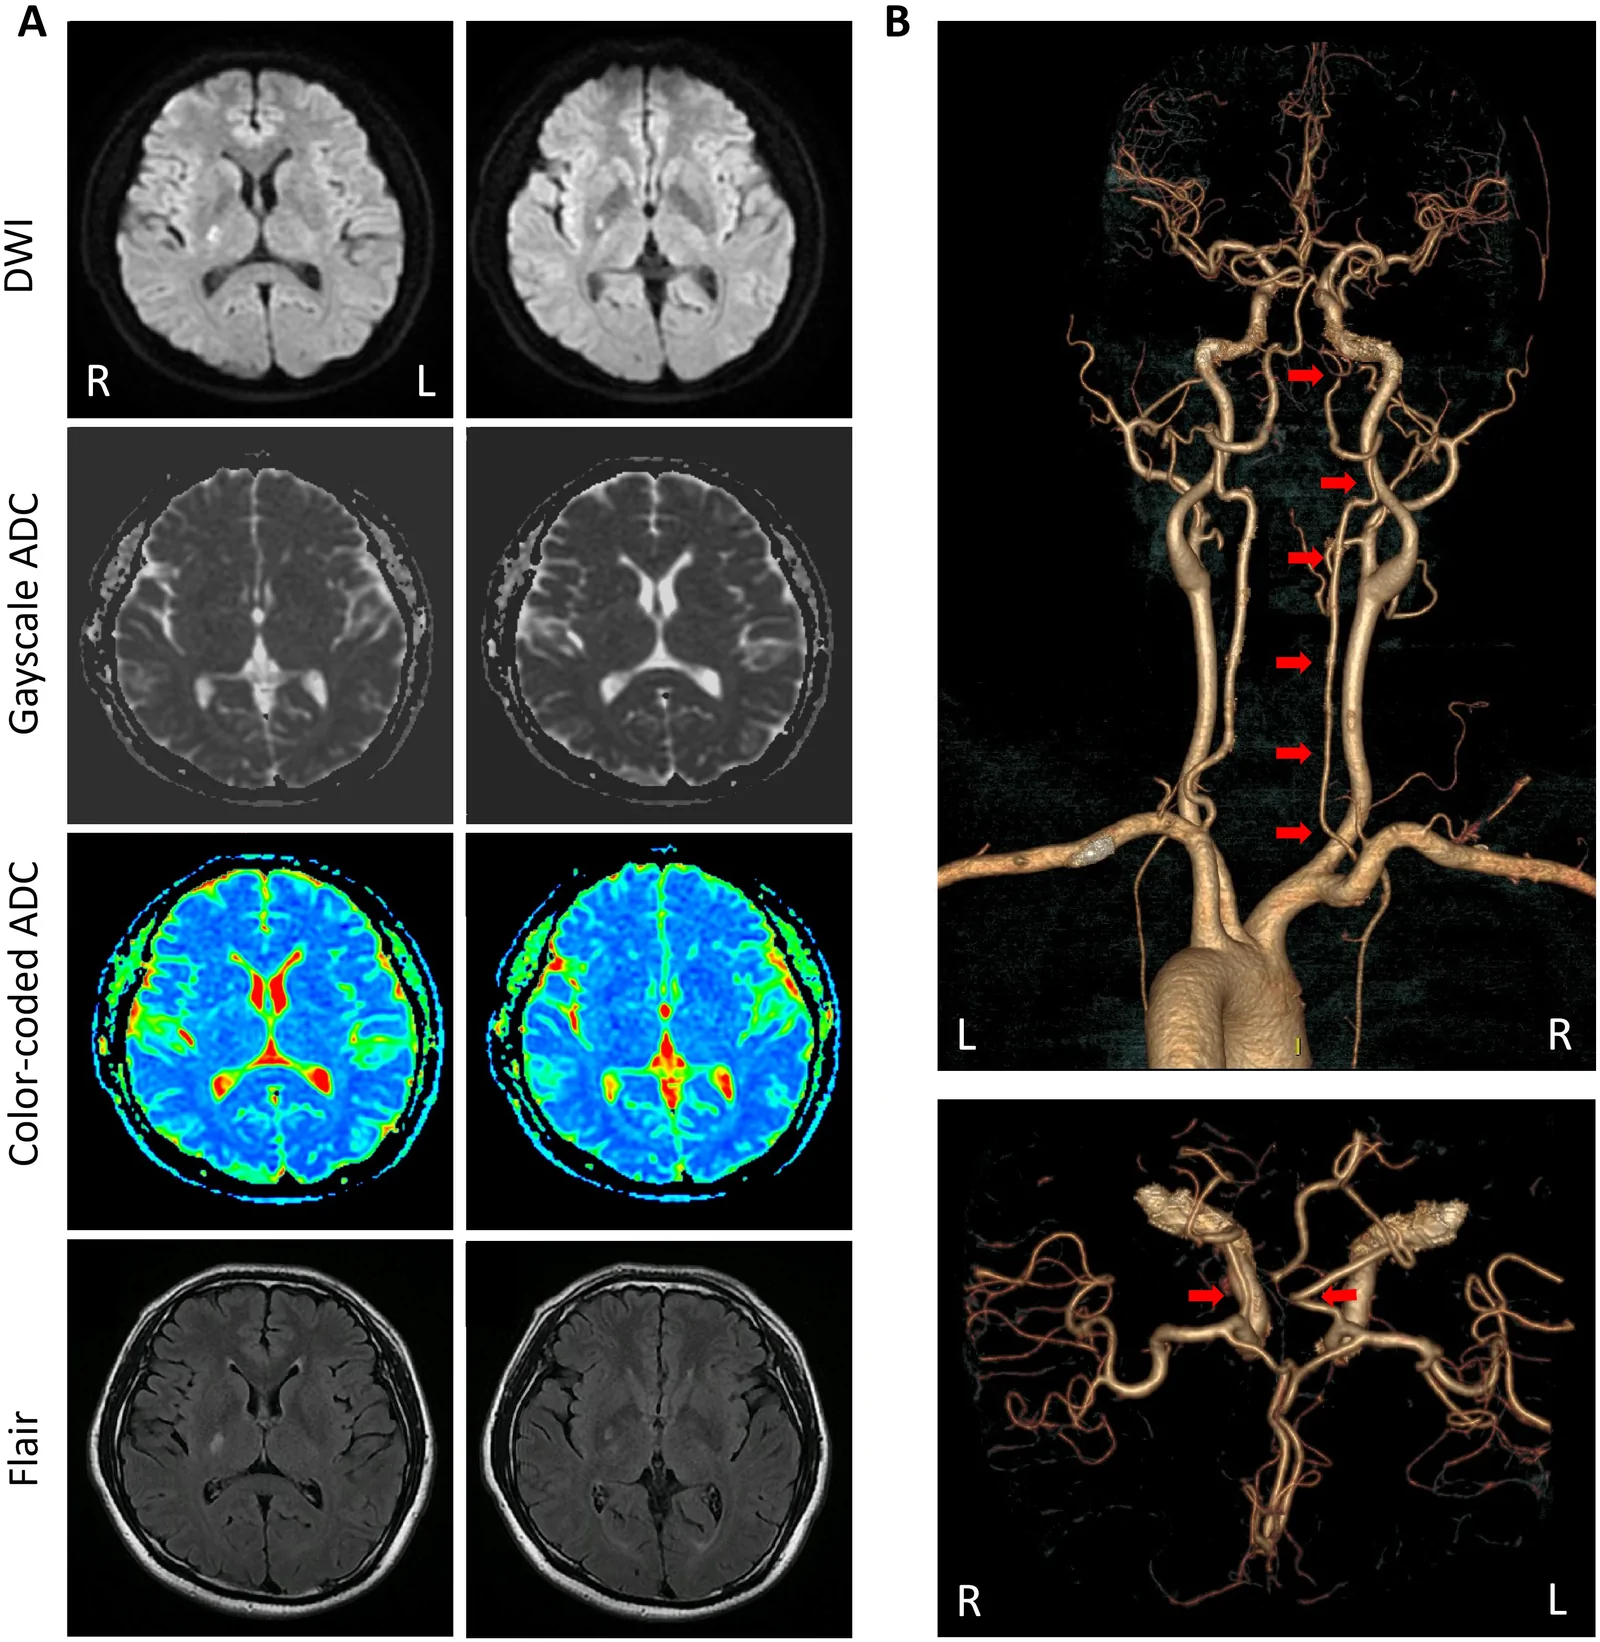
Neuroimaging findings of Case 2. (A) Axial brain MRI sequences demonstrating an acute lacunar infarct in the right globus pallidus and the posterior limb of the internal capsule. The panels are arranged from top to bottom as DWI, grayscale ADC map, color-coded ADC map, and FLAIR, respectively. (B) The CTA of the craniocervical vessels. The upper panel reveals diffuse atherosclerotic changes throughout the anterior and posterior circulations, with notable hypoplasia of the right vertebral artery and complete occlusion at the V4 segment (red arrowhead). The lower panel demonstrates bilateral fetal-type PCAs, indicating a variant posterior circulation anatomy with dominant supply from the internal carotid arteries. DWI, diffusion-weighted imaging; ADC, apparent diffusion coefficient; FLAIR, fluid-attenuated inversion recovery; CTA, computed tomography angiography; PCAs, posterior cerebral arteries.
